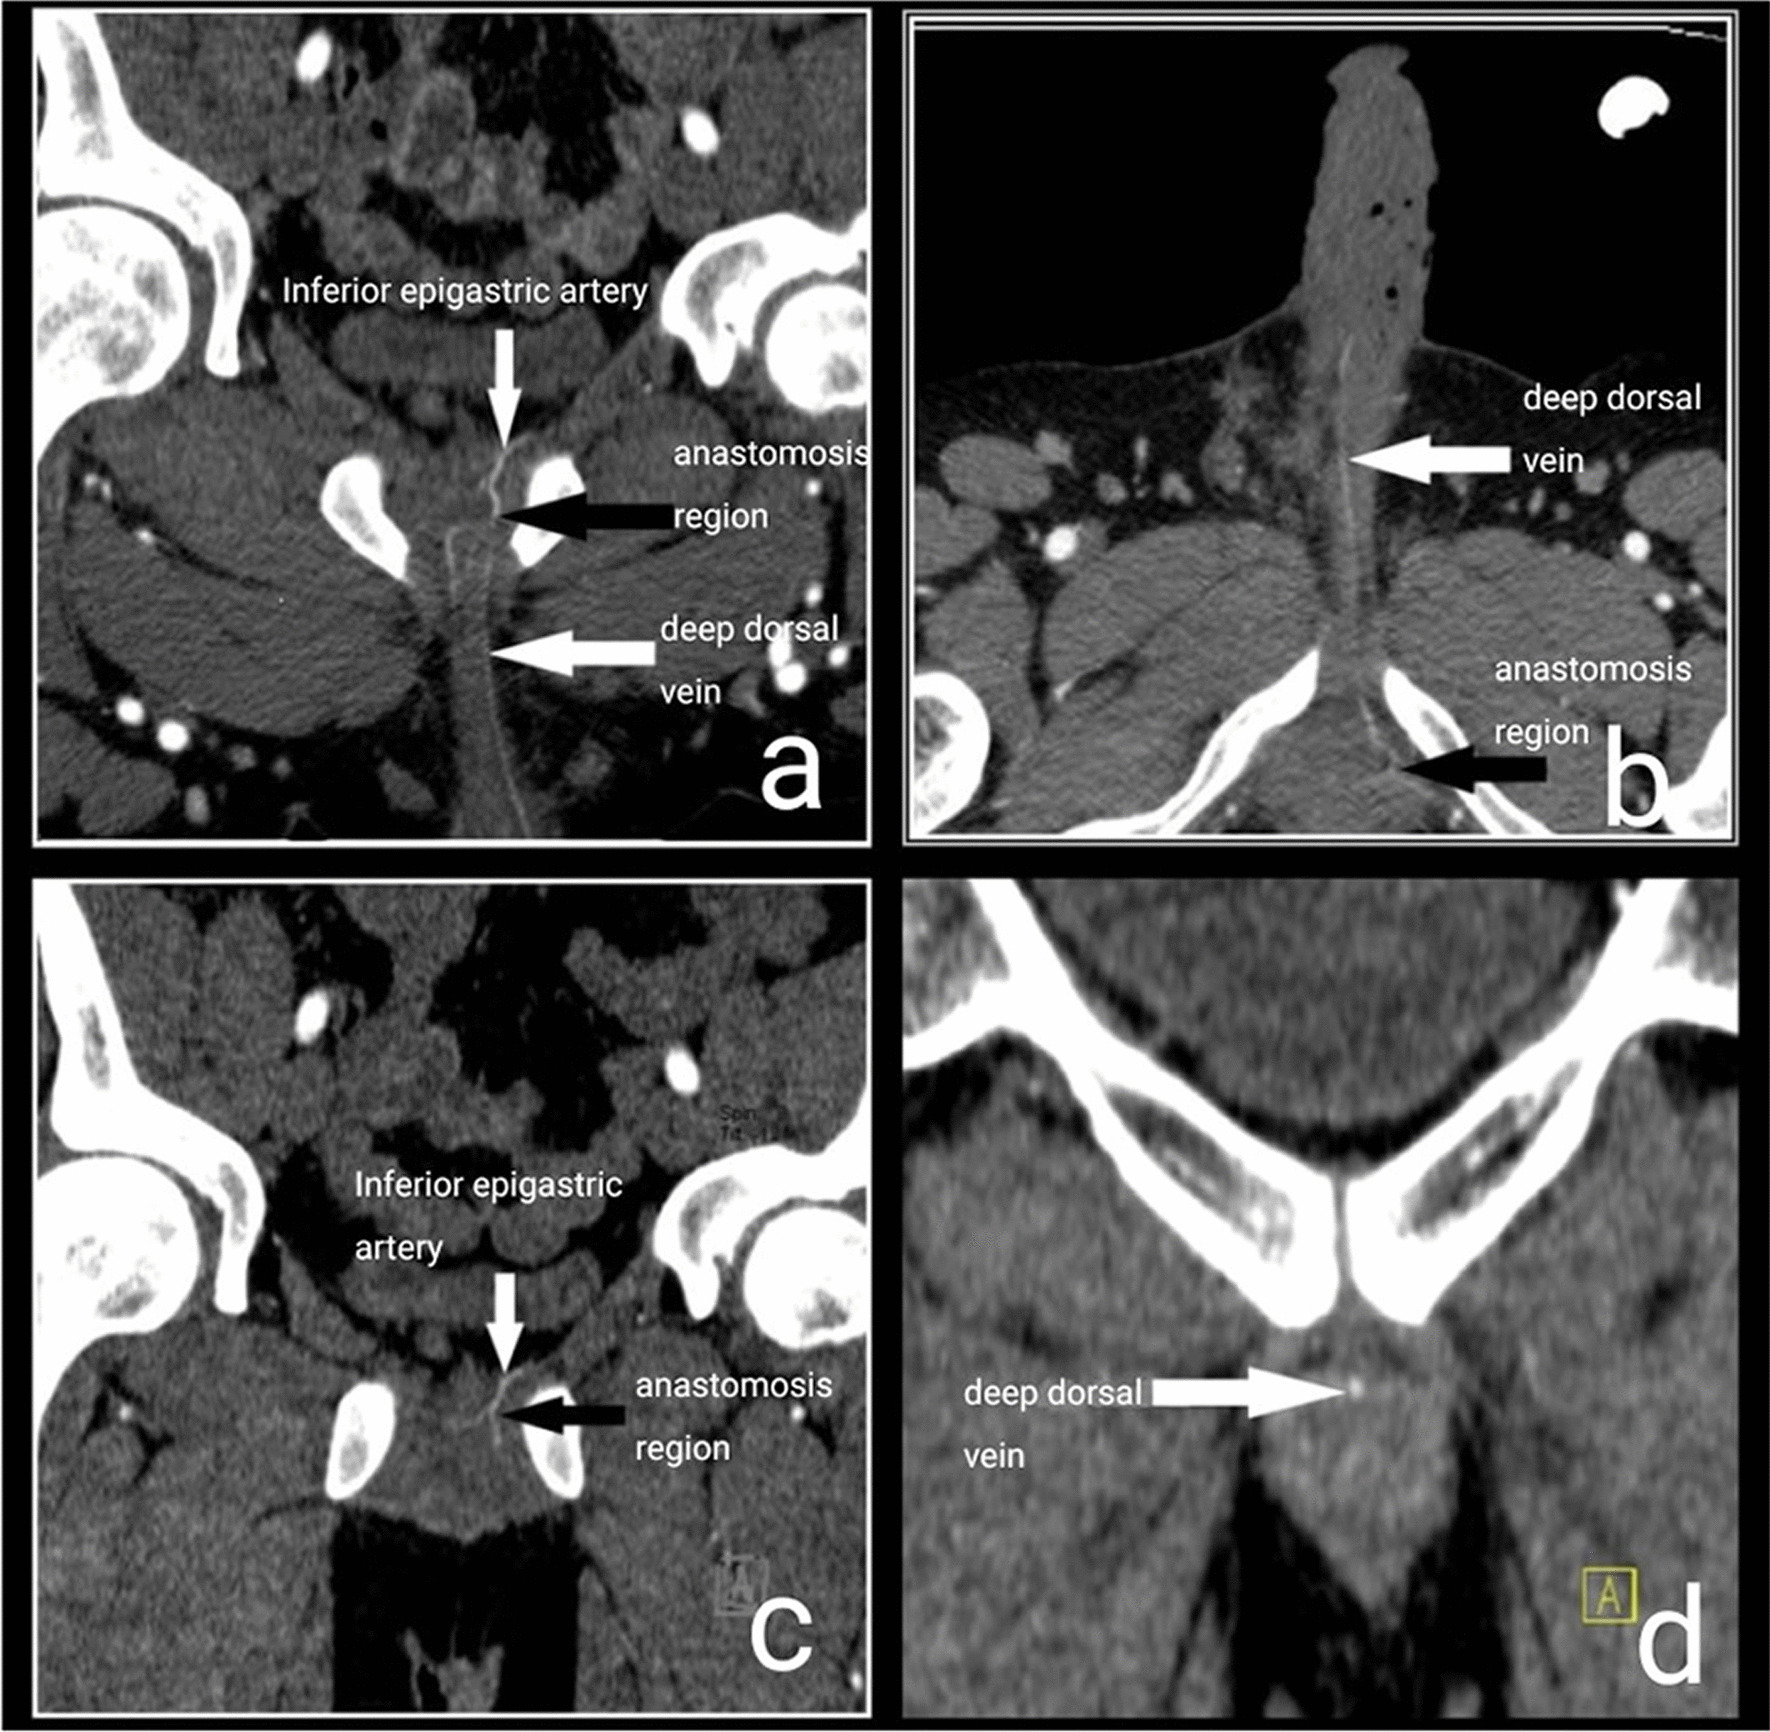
a–d Postoperative pelvic CT angiography images a 30-years-old male. a The image of the left inferior epigastric artery, the anastomotic region, and the arterialized deep dorsal vein and its branch in the coronal cutaway of the penis, b The image of the anastomosis region and deep dorsal vein in the transverse cutaway of the penis, c The image of left inferior epigastric artery and anastomosis region in the coronal cutaway of the penis, d The image of arterialized deep dorsal vein. It is observed that the deep dorsal vein was contrasted in the coronal cutaway of the penis
